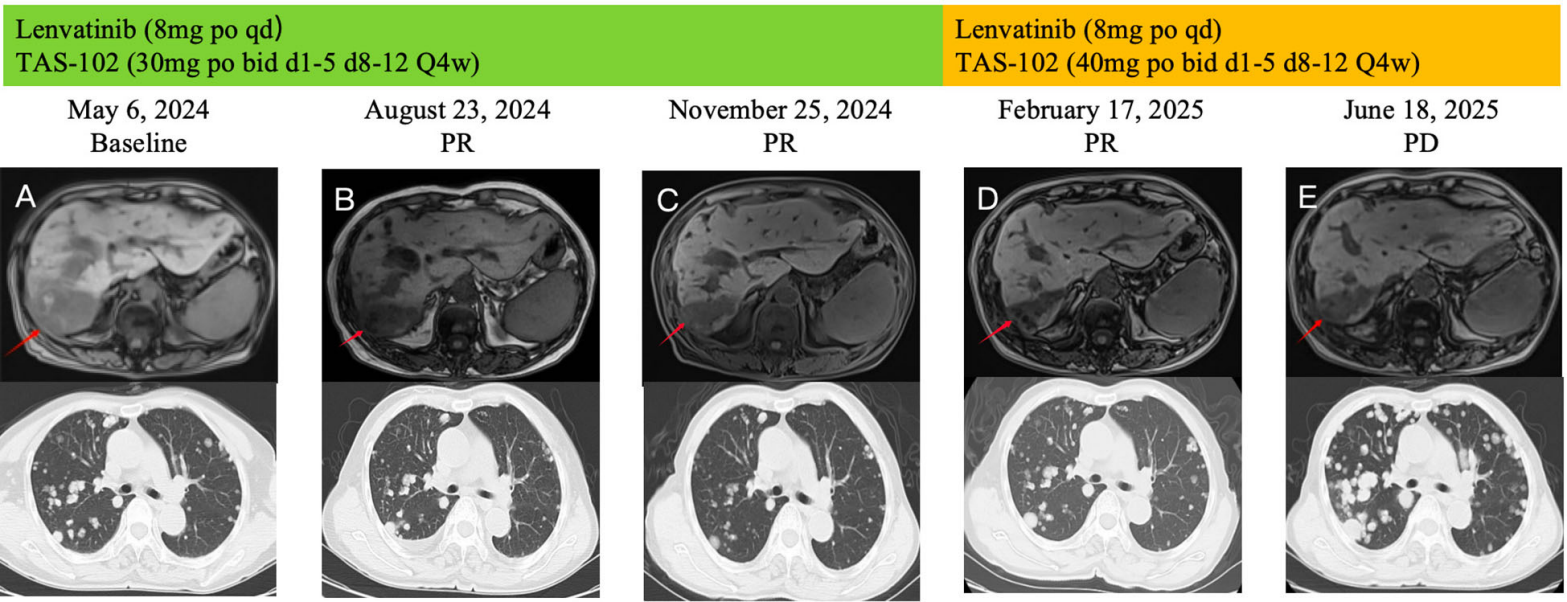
During the course of treatment, follow-up MRI was performed to assess changes in hepatic lesions (A–D) Gradually shrinks. (D, E) Gradually increase), and follow-up CT was performed to evaluate changes in pulmonary lesions (A–C) Gradually shrinks. (C–E) Gradually increase).
